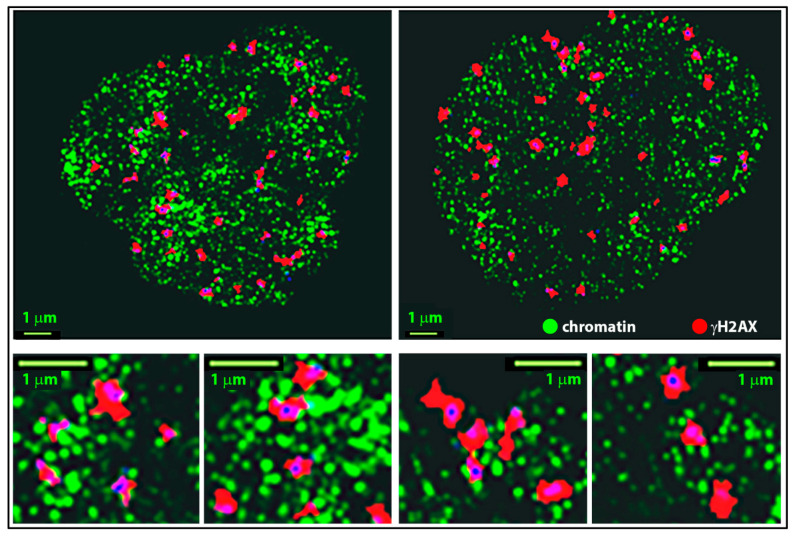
Computed images of two HeLa cell nuclei 30 min after irradiation with 2 Gy X-rays and SMLM of antibody-labeled heterochromatin (green) and γH2AX (red). The artificial images were prepared from the localization matrices (i.e., matrices of signal coordinates and localization errors) obtained for green and red signals. The intensity of the green signals is encoded by the next neighbor density of signals (clustered signals, herein the chromatin domains, are therefore brighter) whereas the red signals are merged into coherent clusters. Blue signals correspond to signal overlap of the two channels. Note that these images were artificially prepared from point data of two color channels by mathematical data processing (see Figure 1 for general description of the whole procedure). Note: Parts of this figure are shown in Figure 1I.
